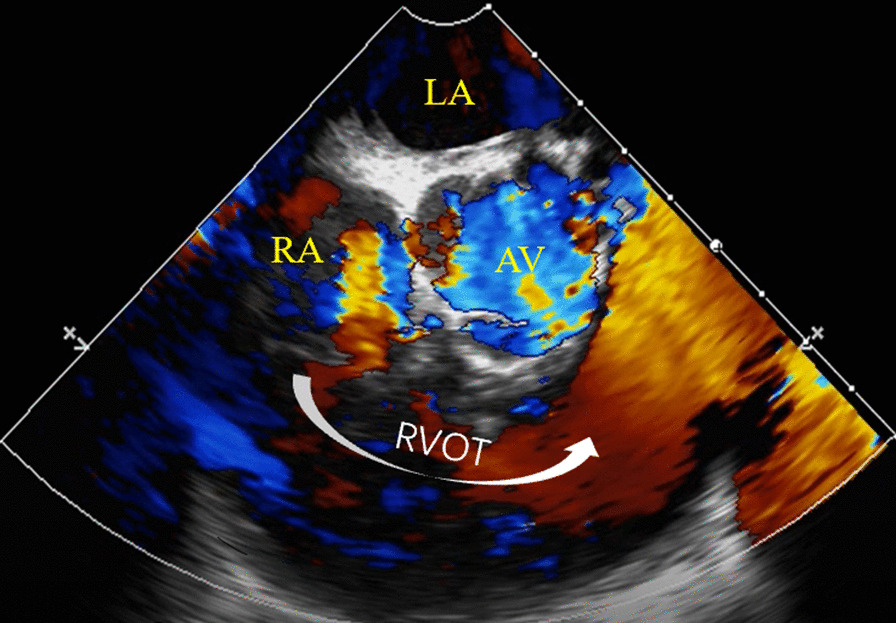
Transesophageal echocardiography (ME RV inflow-outflow tract) after surgery showed the tumor and outflow obstruction disappeared. *LA* left atria, *RA* right atria, *AV* aortic valve, *RVOT* right ventricular outflow tract
